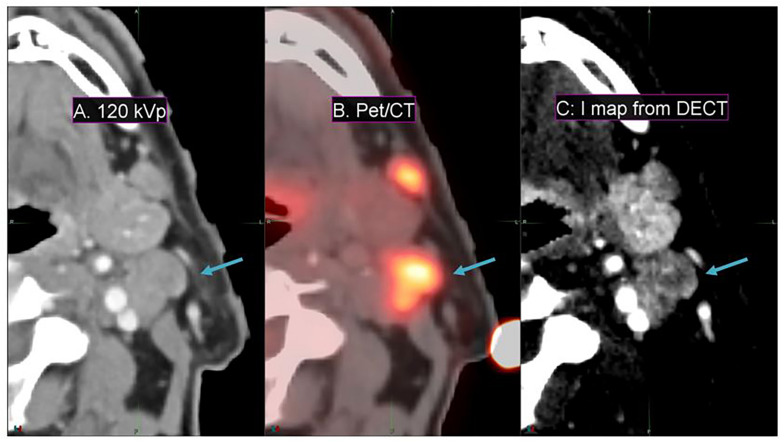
Details from an axial slice of a tonsil squamous cell carcinoma patient imaged with 120-kVp (A), PET/CT (B), and I map derived from a DECT (C). The window width is 400 HU and level is 40 HU. The tonsil tumor is indicated by the arrow.
